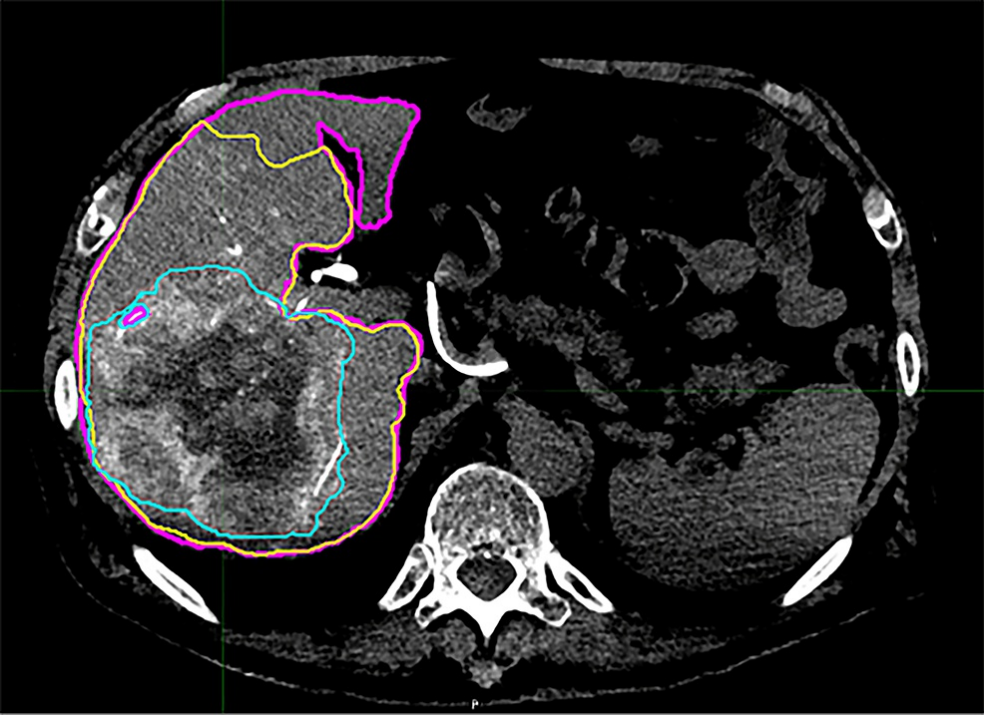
Representative cross-section of operator-defined contours defined on intraprocedural angio-CT Shown are the total liver (magenta), perfused territory (yellow), and tumor (cyan) contours.  The nontumor perfused and unperfused contours are derived from these operator-defined contours by Boolean operation in the software.
